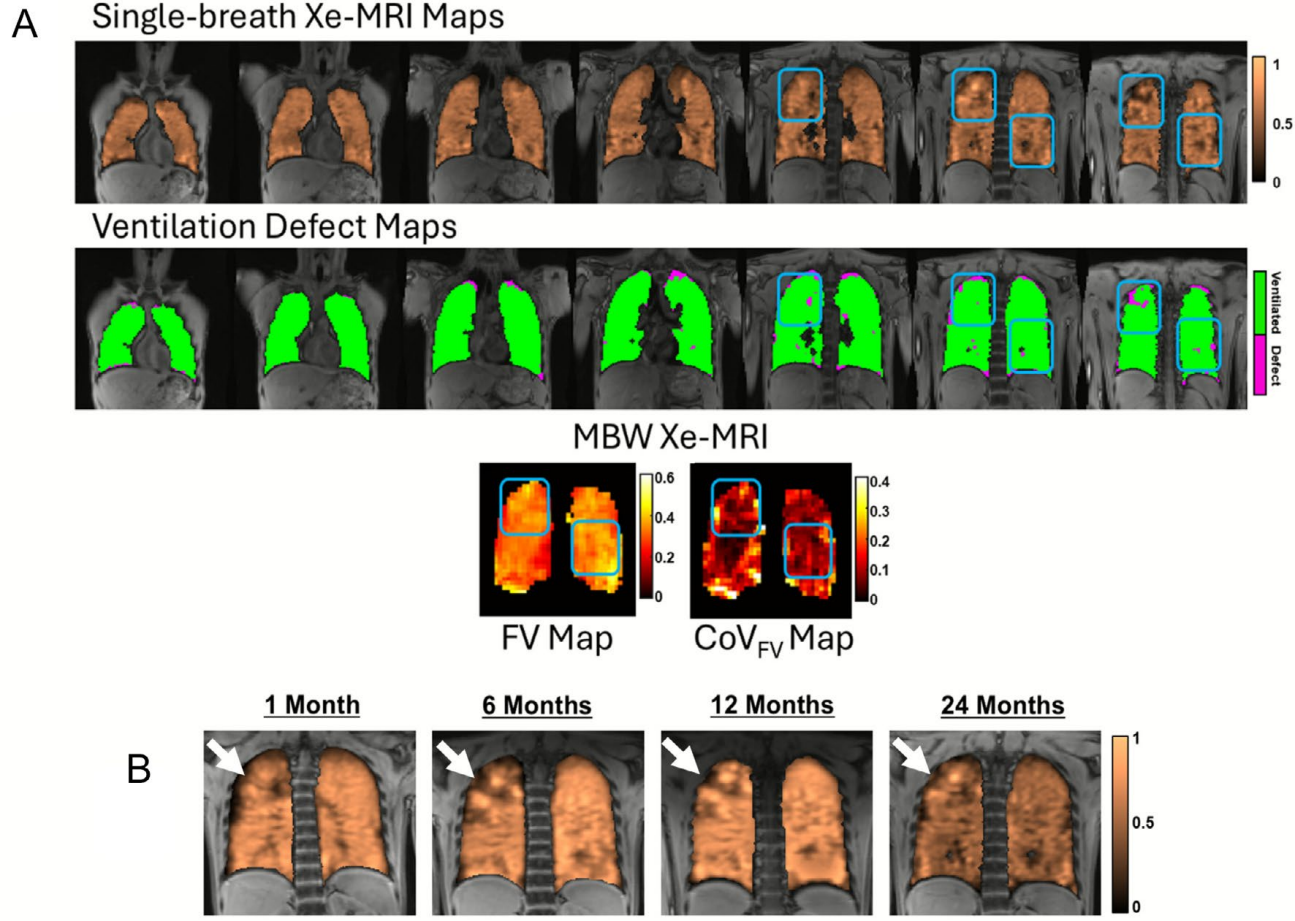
A: Single‐breath Xe‐MRI‐derived ventilation and ventilation defect maps, as well as MBW Xe‐MRI‐derived fractional ventilation (FV) and coefficient of variation of FV (CoV_FV_) maps for the 24‐month visit of the 14‐year‐old male participant in Figure 3A. Blue regions highlight visible heterogeneity in the ventilation maps that do not appear in the defect maps, but appear in FV and CoV_FV_ maps. B: Single‐breath Xe‐MRI‐derived ventilation maps for the same participant across all visits. The area of compensatory ventilation (white arrows) developed after 1 month and remained persistent up to 24 months.
